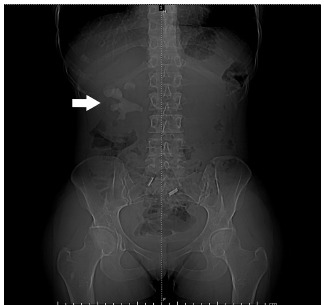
Computed tomography scout image demonstrating large staghorn calculus (arrow).
